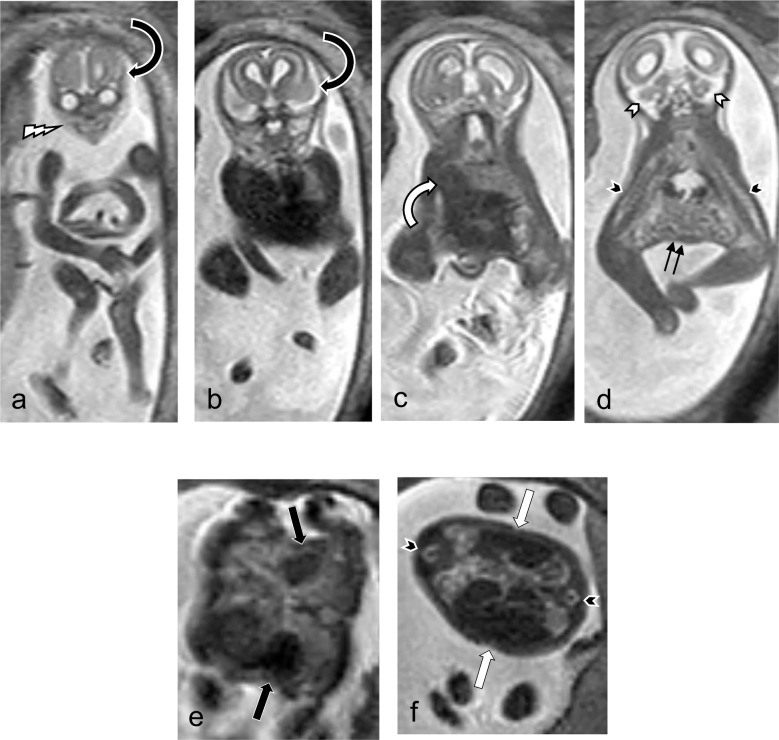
Fetal MRI of cephalopagus at gestational age 18 weeks, 3 days, showing ventral fusion of the head through the abdomen. a-d T2-weighted SSH TSE sequential ventral-to-dorsal coronal images show fused anterior brain (*curved black arrow*), single face (*white lightning bolt*), herniation of one liver through a congenital diaphragmatic hernia (*curved white arrow*), separate cerebellums (*white arrowheads*) and spinal canals (*black arrowheads*), and bowel in a shared peritoneal cavity (*double small black arrows*). e T2-weighted SSH TSE axial image through the conjoined chest shows separate hearts (*black arrows*). f T2-weighted SSH TSE axial image through the conjoined abdomen shows separate livers (*white arrows*) and spinal canals (*black arrowheads*). *SSH TSE*, single-shot turbo spin-echo
